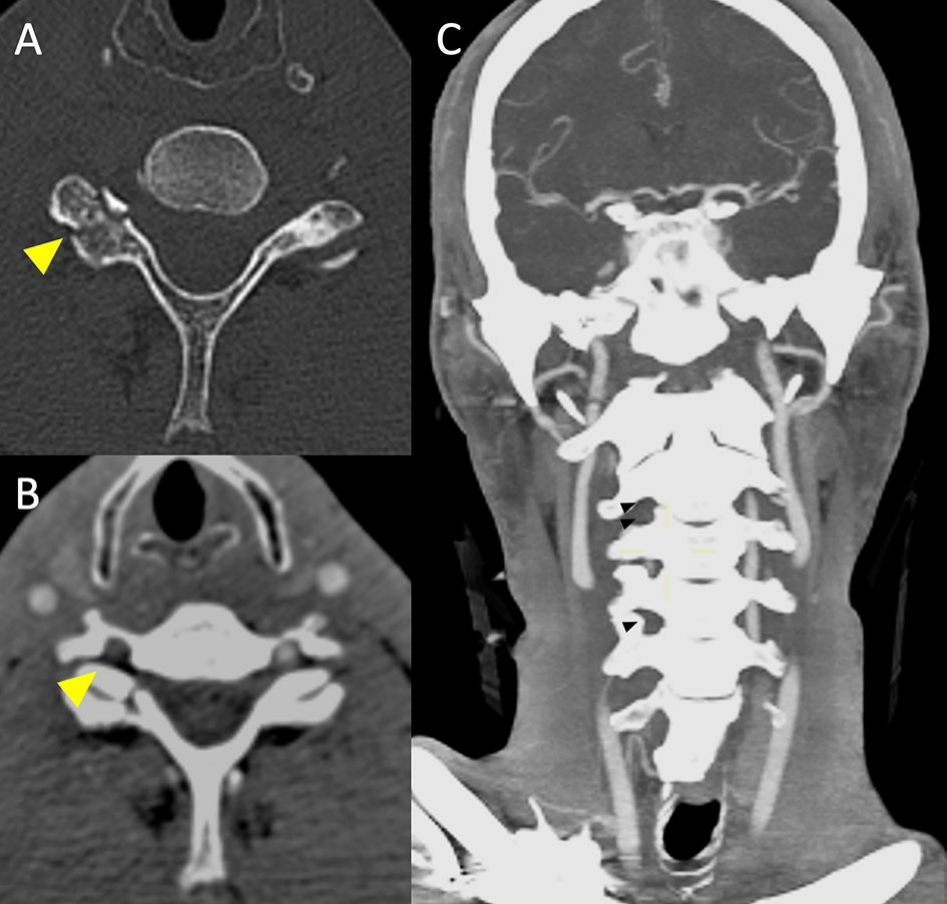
Basal CT scan of a patient in the early fifth decade of life following a motor vehicle accident. Non-enhanced CT scan (A) demonstrates a fracture of the right superior articular process of the C6 vertebra (yellow arrowhead). CTA (B, C) shows lack of opacification of the right vertebral artery in the transverse foramen (B, yellow arrowhead), starting from the distal V1 segment (not shown), continuing along part of the V2 segment (C, black arrowheads) and V3 segment and ending at the proximal V4 segment (not shown), consistent with arterial dissection with subsequent occlusion of the vessel (Denver Grade IV)
